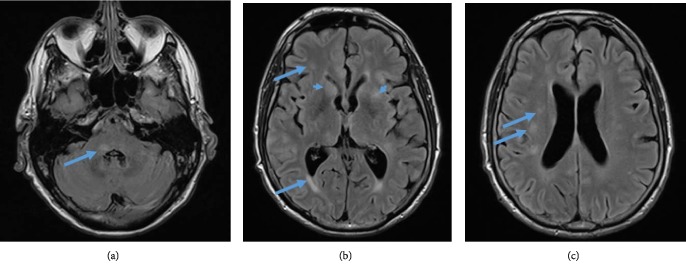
Initial MRI brain, FLAIR transversal images with multifocal abnormal hyperintensities (arrows). (a) Middle cerebellar peduncle. (b) Periventricular and subcortical white matter, right caudate nucleus, and left lentiform nucleus. (c) Deep white matter.
